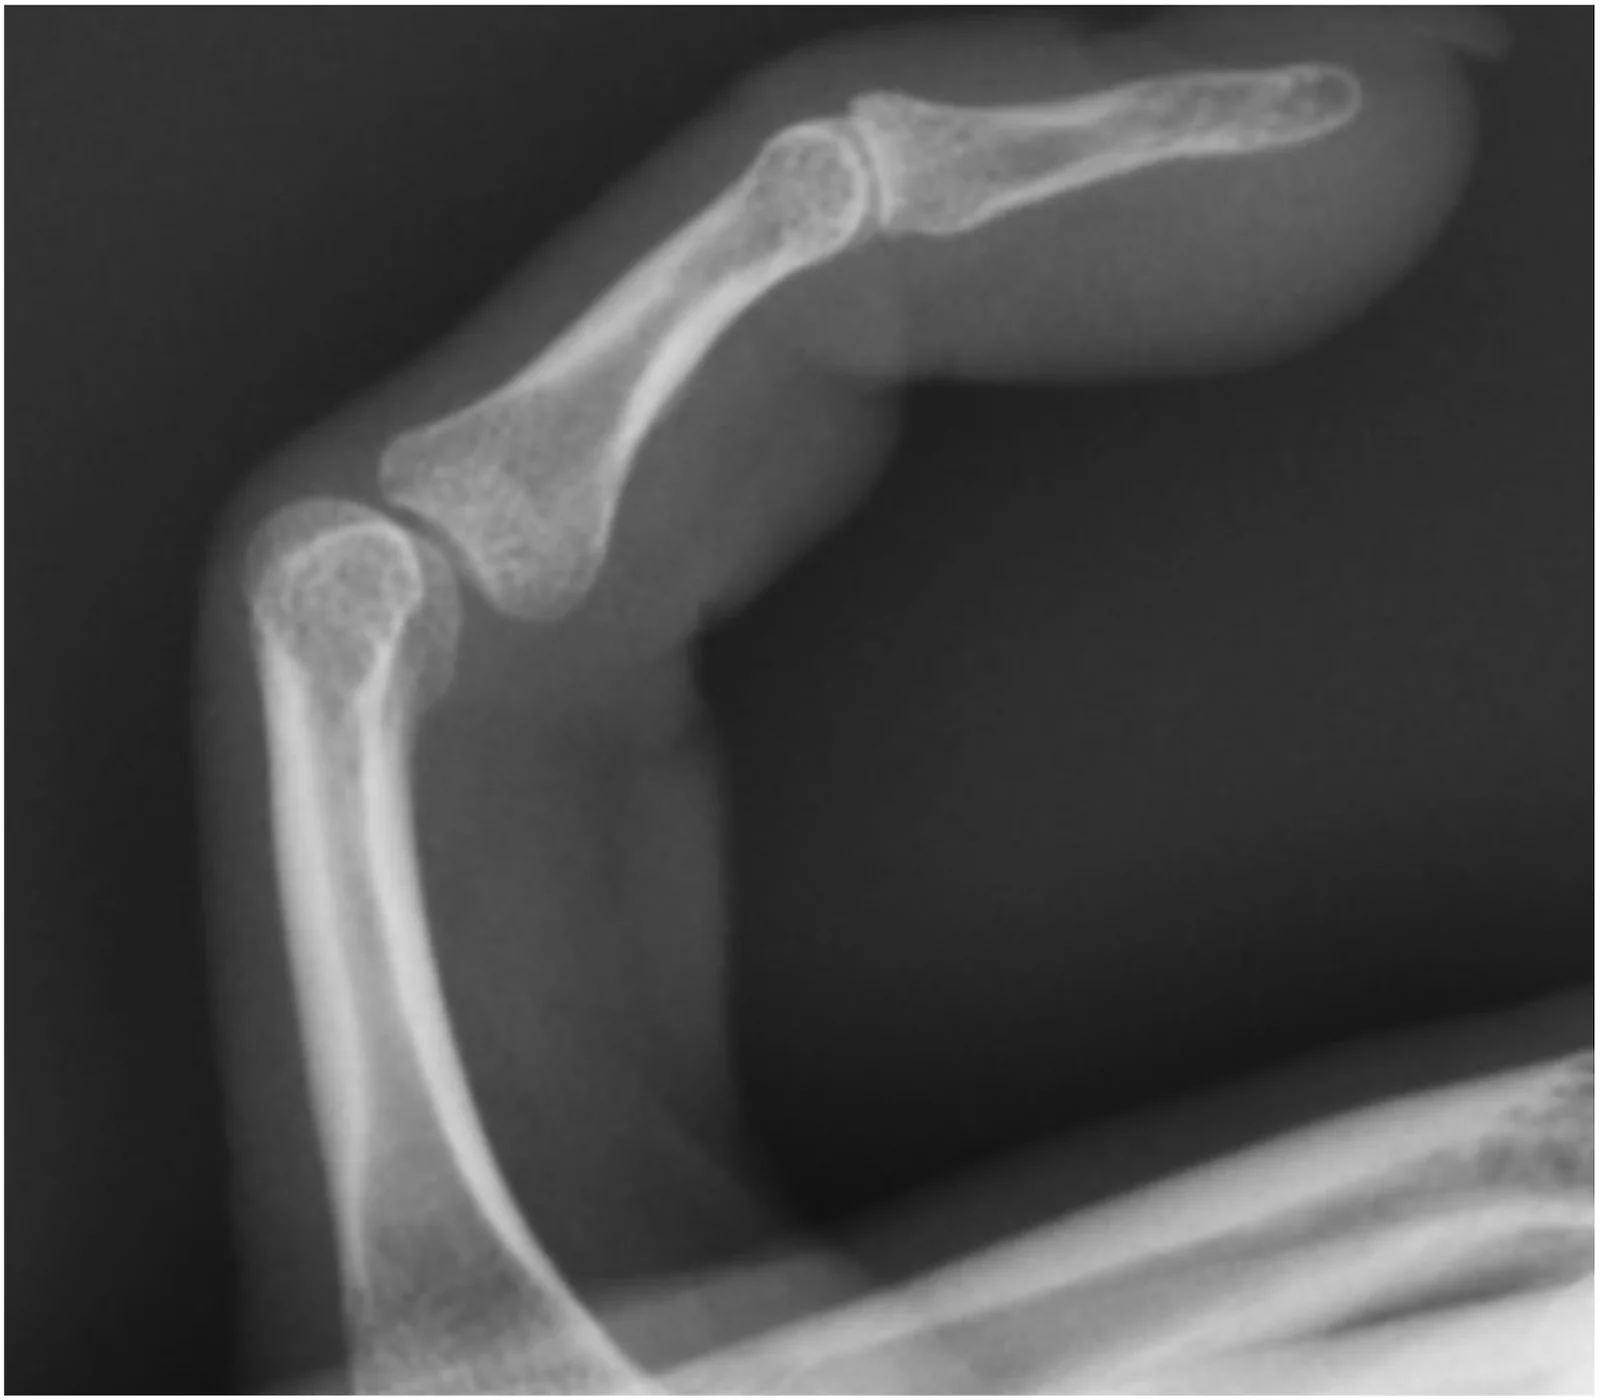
Lateral radiograph of the left little finger demonstrating PIP joint flexion with preserved joint congruency.
